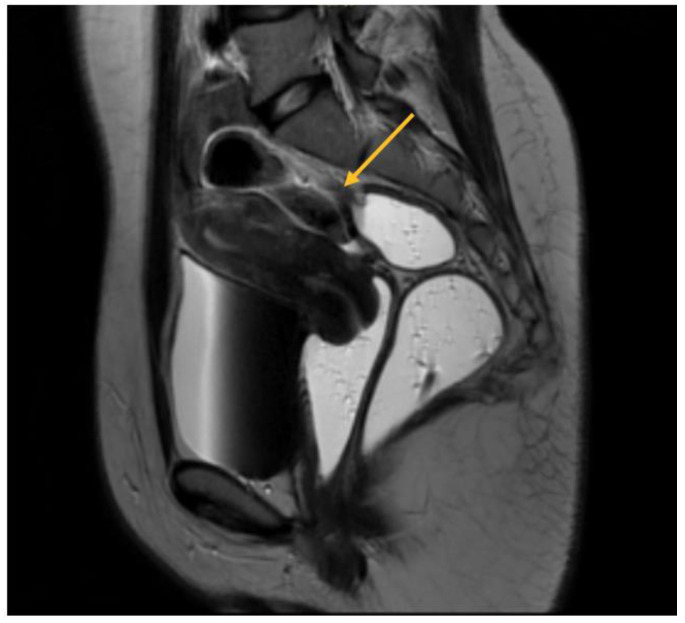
MRI aspect on T2 sagittal: parametrial endometriosis nodule.
